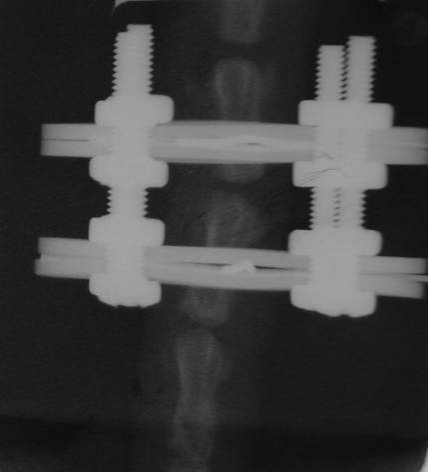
An x-ray showing the accurate position of the wires through the vertebral bodies and the angular deformity of the intervertebral disc.
